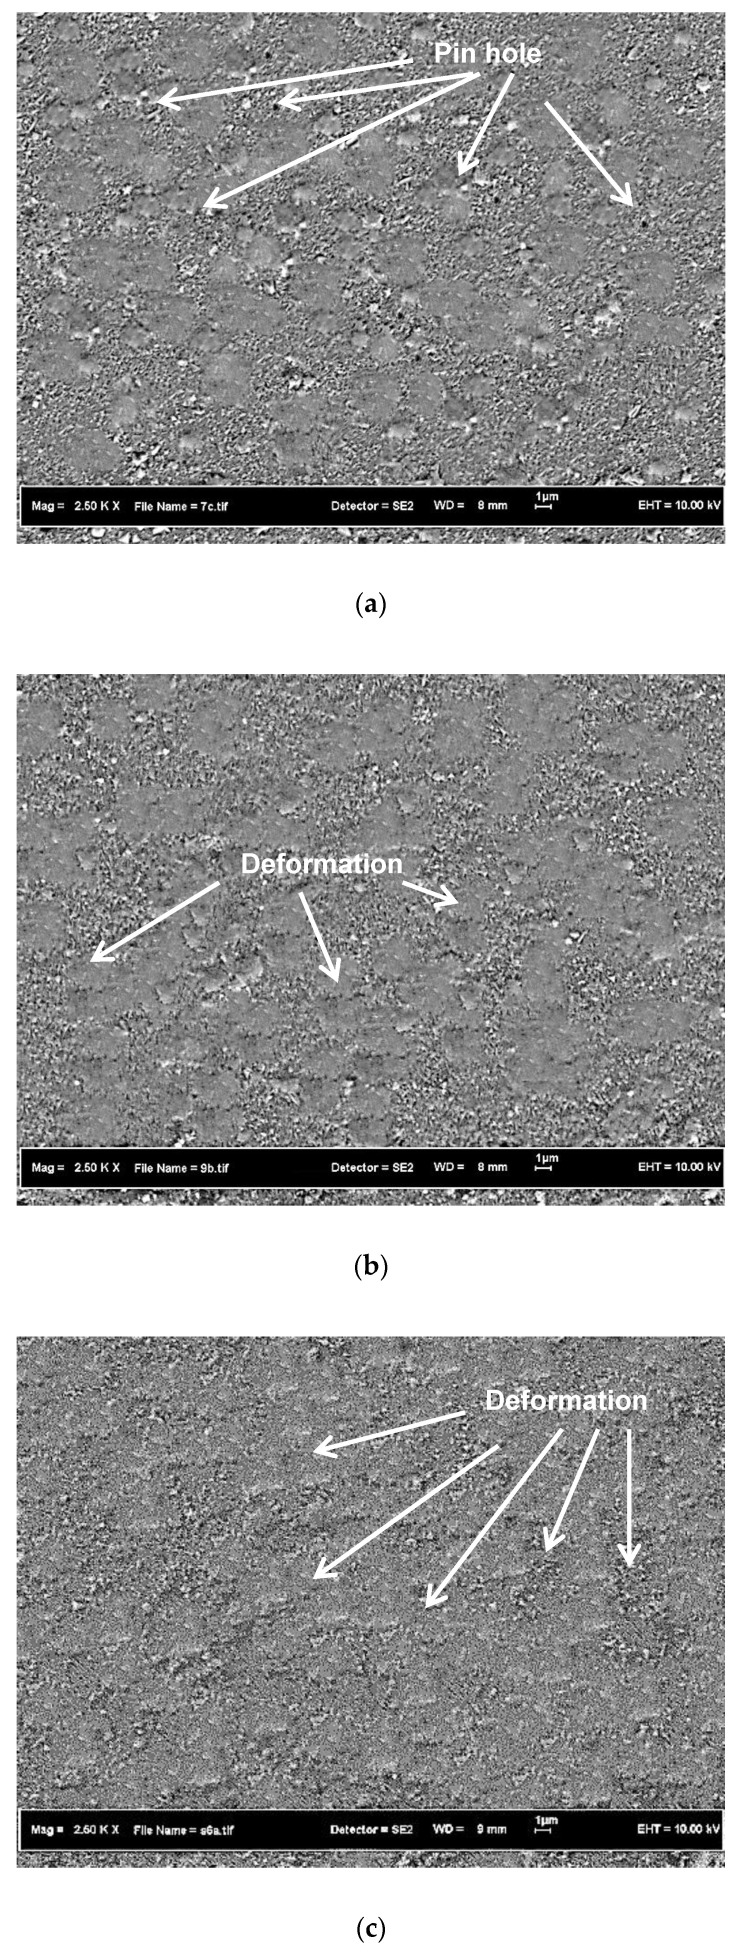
Surface morphology of TiN after ultrasonic treatment at 16 kHz for exposure times of (a) 5 min, (b) 8 min, and (c) 11 min.
